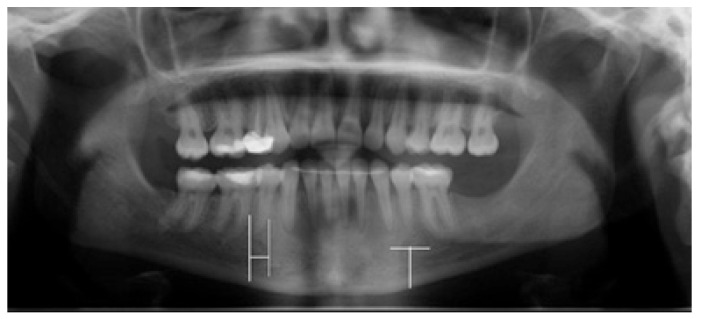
Panoramic radiography. On the right we observe the technique used for the measurement of the mental loop. On the left side we observe the technique used for the measurement of the distance between the mental foramen and the lower border of the mandible.
